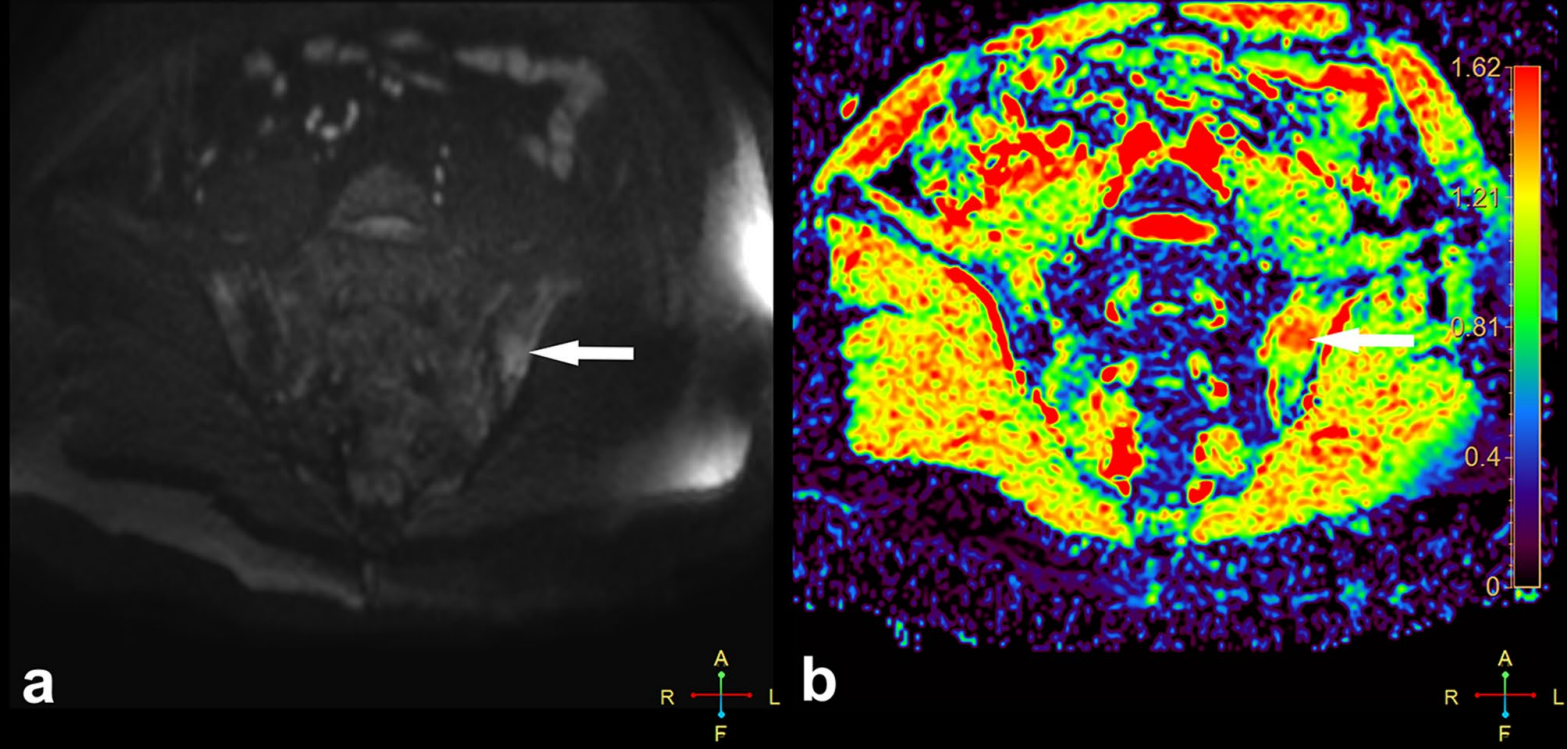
Active inflammatory lesion in the course of axSpA (white arrow) located in the iliac part of the left sacroiliac joint on DWI (*b* = 800) sequence (a) and colour ADC map (b). DWI, diffusion-weighted imaging; ADC, apparent diffusion coefficient
